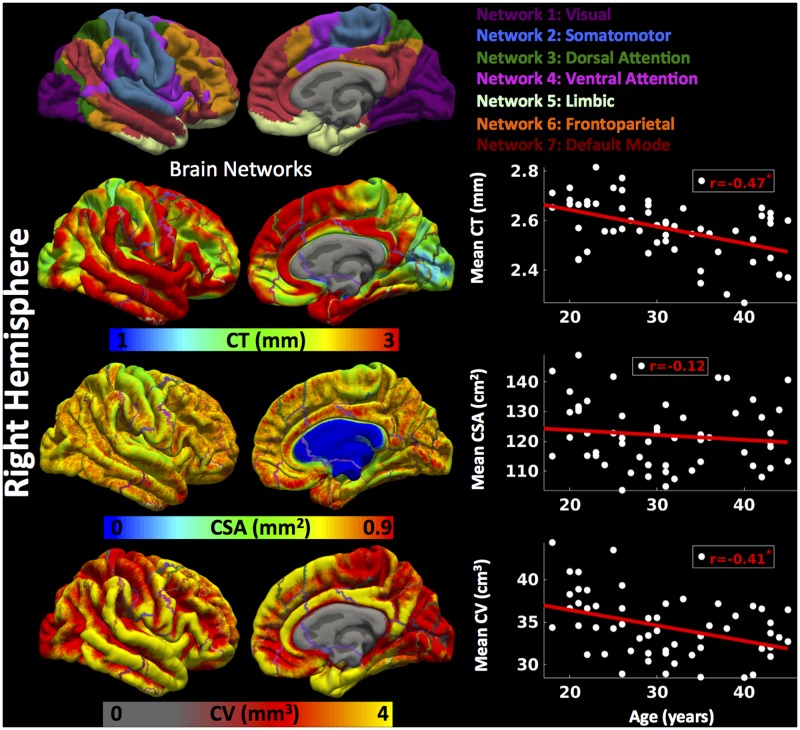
Brain parcellation and age-related changes in mean cortical measures for right hemisphere. Right hemispheric brain parcellation into seven functional networks and vertex-wise maps for cortical thickness (CT in mm), cortical surface area (CSA in mm^2^) and cortical volume (CV in mm^3^). Linear plots showing age-related significant reduction in mean CT (in mm), non-significant reduction in mean CSA (cm^2^) and significant reduction in mean CV (cm^3^) over right hemisphere.
